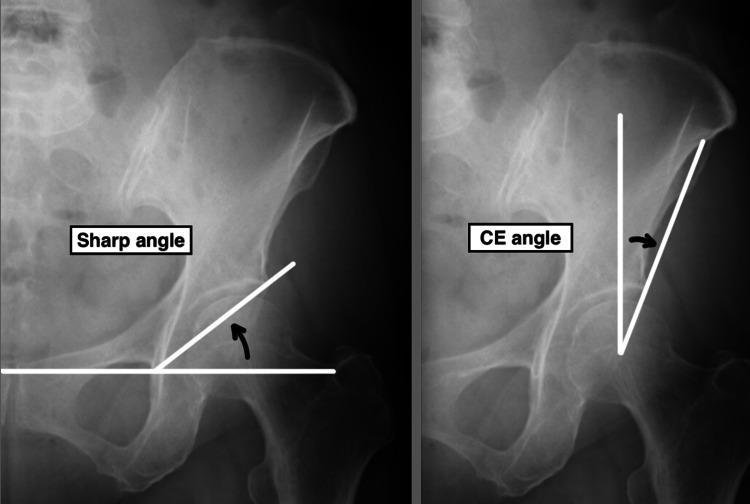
X-ray image of the hip joint, coronal plane CE: center-edge
